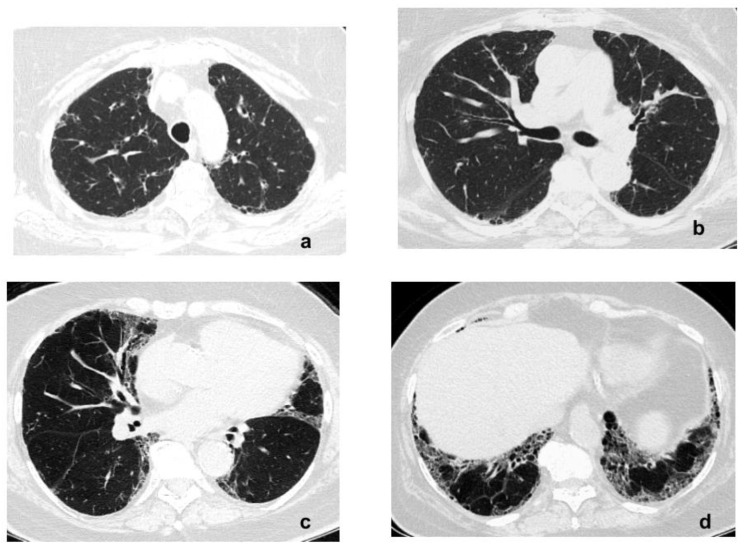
(a–d) HRCT images (2016) show no progression of interstitial lung fibrosis.
